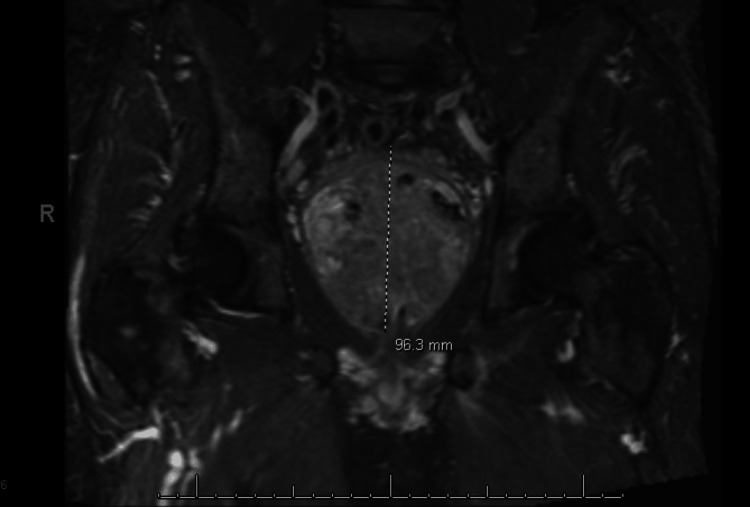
T2 STIR coronal MRI of the pelvis without contrast revealing prostatomegaly measuring 9.5 x 8.3 x 9.6 cm, with heterogeneous appearance. STIR: short tau inversion recovery
